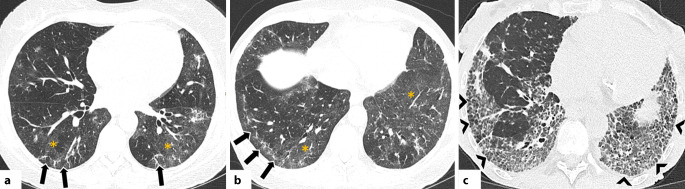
a, b Axiale hochauflösende Computertomographie (HRCT) zeigen bei einer Patientin mit einem Antisynthetase-Syndrom (Anti-Jo-1-Antikörper) diffuse, zentral betonte Milchglasverschattungen (*gelbe Sternchen*) sowie periphere lineare Verdichtungen in den dorsalen Abschnitten der Oberlappen und des rechten Unterlappens (*schwarze Pfeile*), hinweisend auf ein perilobuläres Verteilungsmuster. Diese Befunde sind vereinbar mit einem kombinierten NSIP-/OP-Muster (nichtspezifische und organisierende Pneumonie). c Zehn Jahre später zeigt die Verlaufskontrolle eine Progredienz zu einem UIP-Muster (gewöhnliche interstitielle Pneumonie) mit Milchglasverdichtungen (hier Hinweis auf feine Fibrose), Traktionsbronchiektasen und bilateralem subpleuralem Honeycombing (*schwarze Pfeilköpfe*) in den Mittel- und Unterlappen; zudem deutlicher Volumenverlust beider Unterlappen
